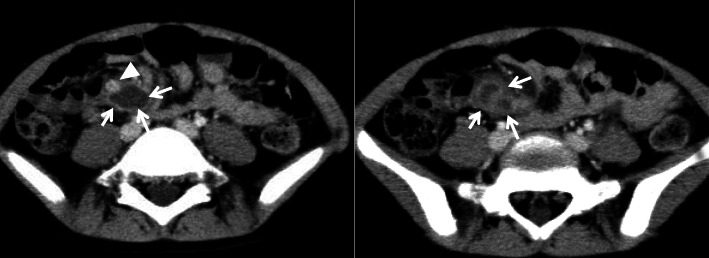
Follow-up CT. On the follow up CT at 3 months revealed, decrease in size of the lesion is seen. There is a enhancing nodule seen in the cystic wall (arrow)
